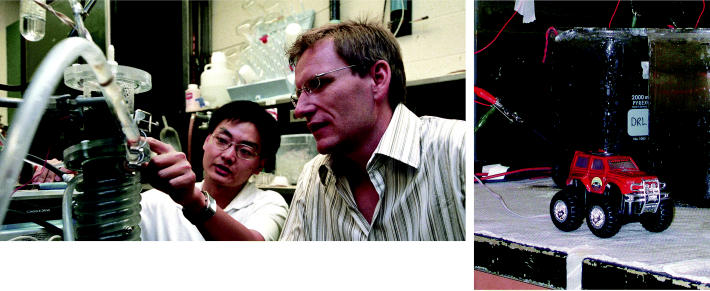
Big plans for small microbes. Jason He (left) and Lars Angenent inspect their MFC. In Derek Lovley’s lab (right), a model SUV is powered by marine geobatteries.
